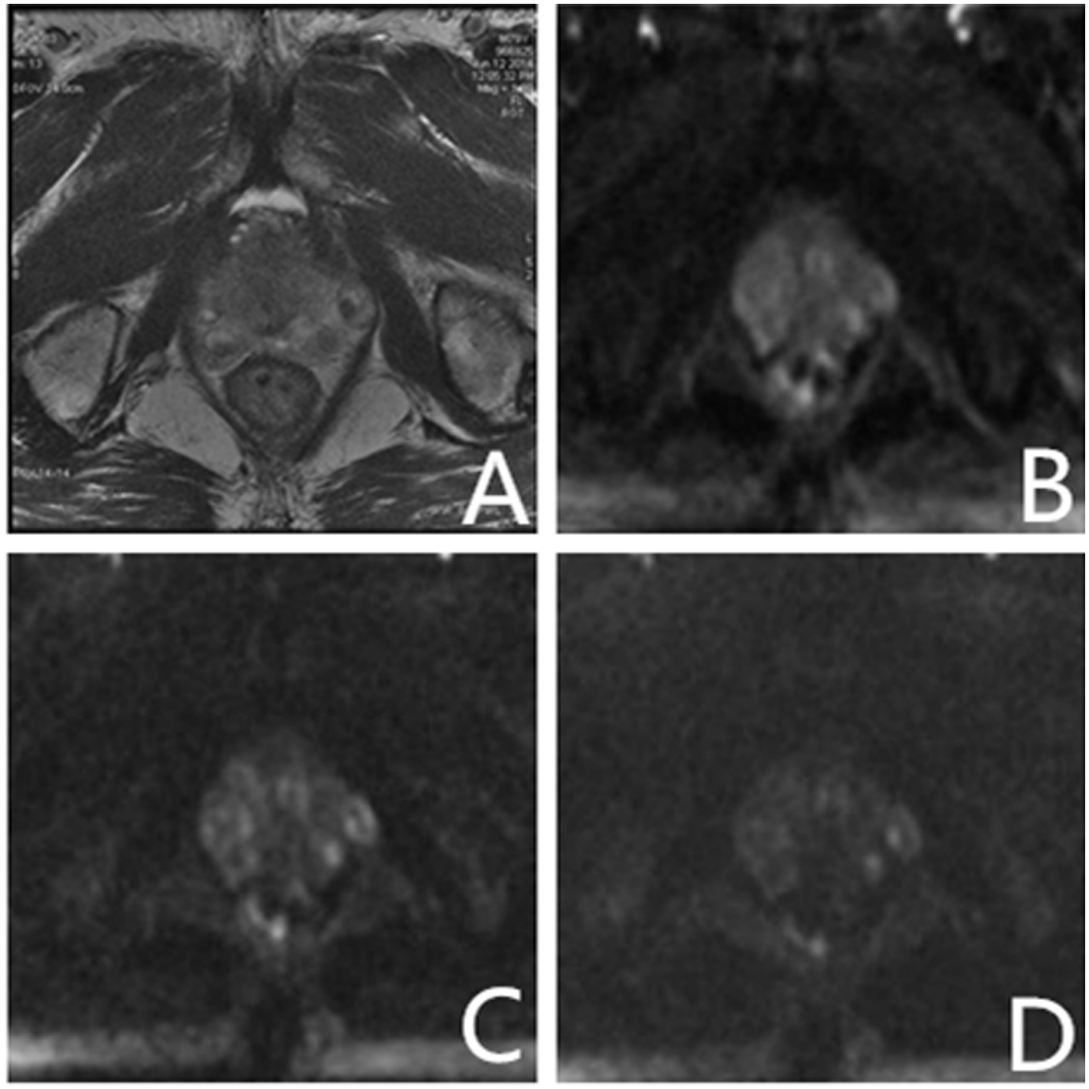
Images from a 79 yr old male patient with total PSA 6.1 ng/ml. Systematic transrectal biopsy confirmed prostate hyperplasia and prostatitis. (A) High-solution T2-weighted imaging shows low signal lesions on bilateral peripheral zone; (B–D) On conventional and ultrahigh b-value diffusion-weighted imaging (b = 1000, 2000, 3000 s/mm^2^), bilateral PZ lesions showed slightly higher signal nodules. Intensity of lesion signals decreased with increase in b-value, whereas the intensity of normal tissue signals reduced significantly. Key: PSA—Prostate specific antigen; PZ—Peripheral zone.
